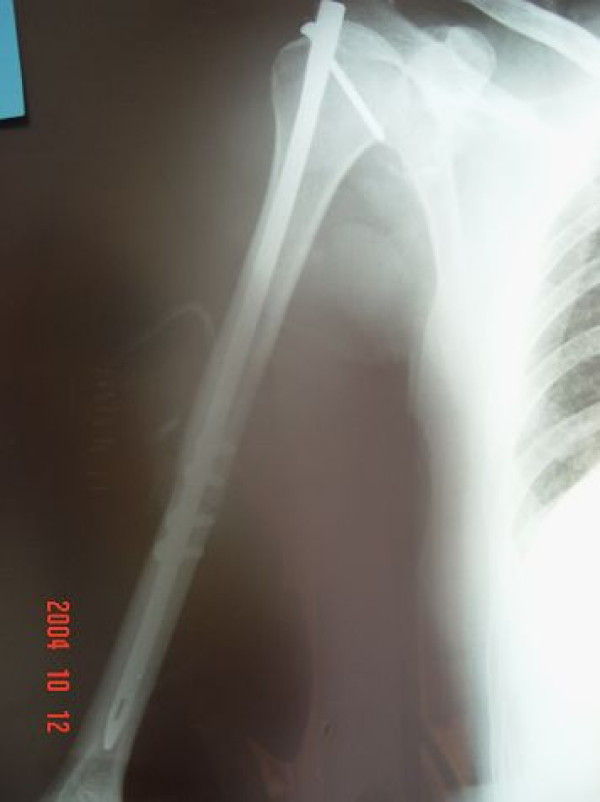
Anteroposterior radiograph after internal fixation of the shaft fracture and reduction of the dislocation.
